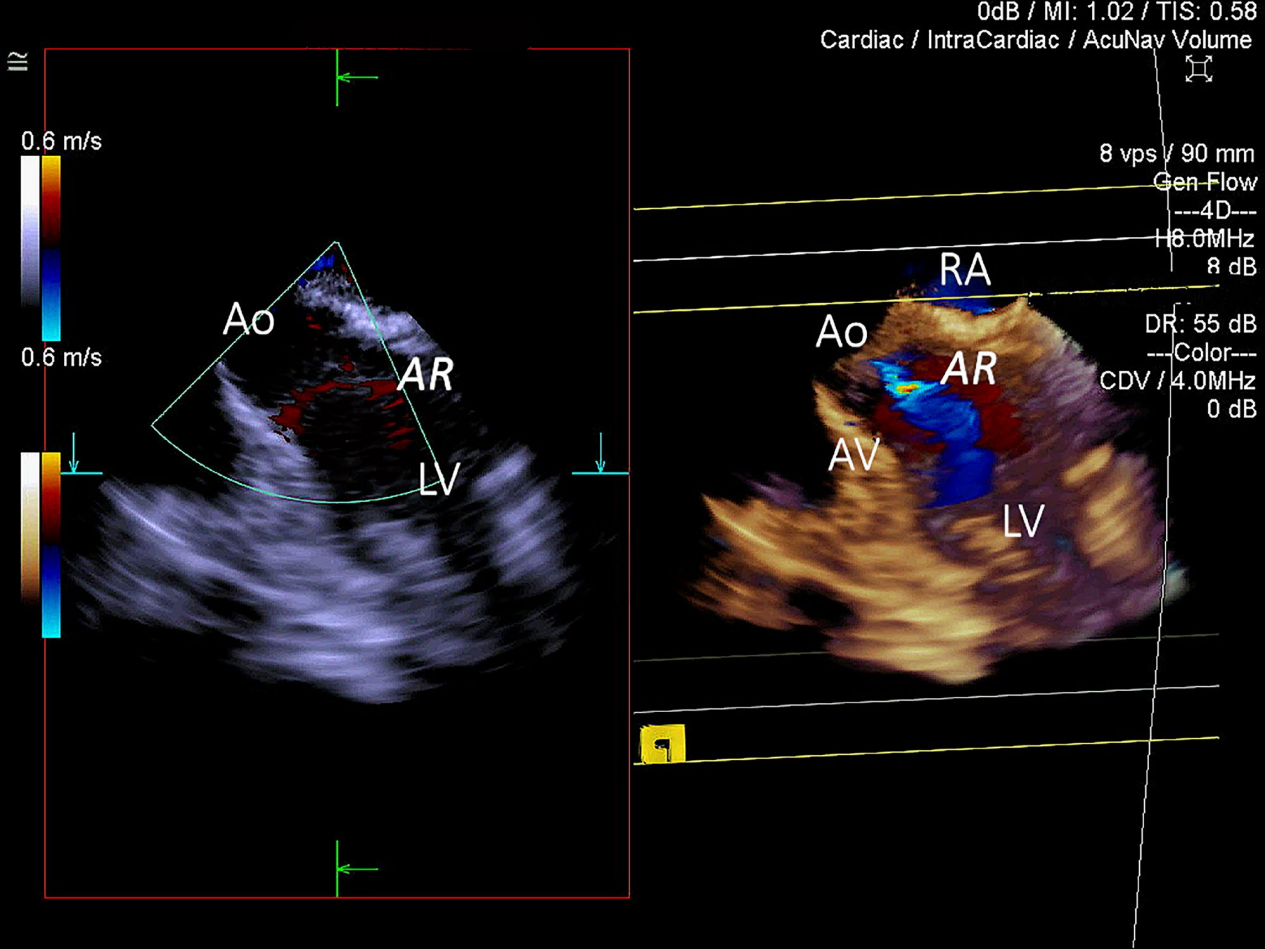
3D ICE Colour Doppler (CD) assessment of the aortic valve for pre-existing pathology. Left side of the image—a cut plane equivalent to the 2D ICE long-axis view of the left ventricle including left ventricular outflow tract, aortic valve and aortic root. Right side of the image—3D CD volume with default cut plane activated. 2D cut plane failed to detect aortic valve insufficiency. *RA* right atrium, *Ao* ascending aorta, *AV* aortic valve, *LV* left ventricle, *AR* trivial aortic regurgitation identified before implantation of the Impella.
